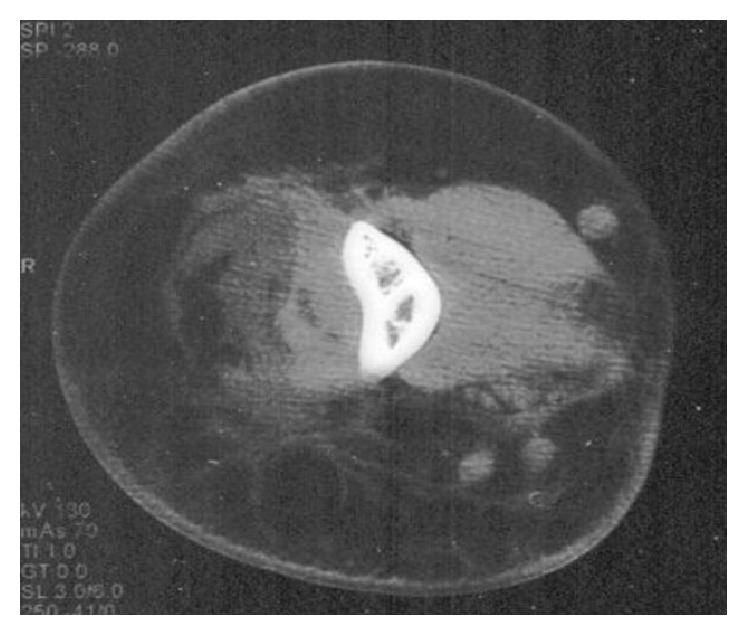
CT scan of left arm: fluidic collection with heterogeneous rearrangement of the fatty tissue with septa thickened and presence of muscular necrosis.
